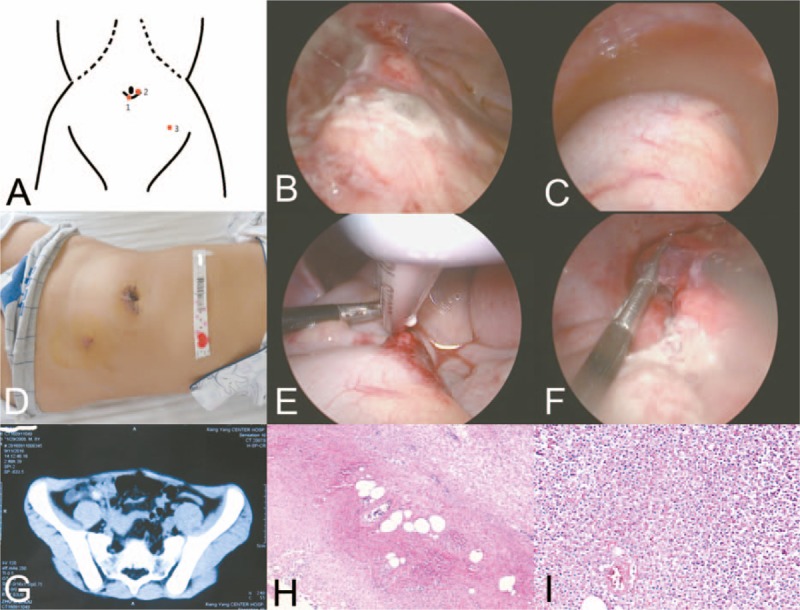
The figure of operation-correlated and examination. (A) The position of 3 trocars are shown with only 2 incisions. (B, C) Results of diagnostic laparoscopy, many exudates, and pus are seen in abdomen; the 2-incision wound healed, as shown in (D) showed. (B) and (C) indicates a clear view and minimal bleeding perioperation; a CT scan revealed an enlarged appendix, thickened wall, and appendiceal fecalith as shown in (G). (H, I) A pathological diagnosis of an acute phlegmonous appendicitis with periappendicitis is indicated.
